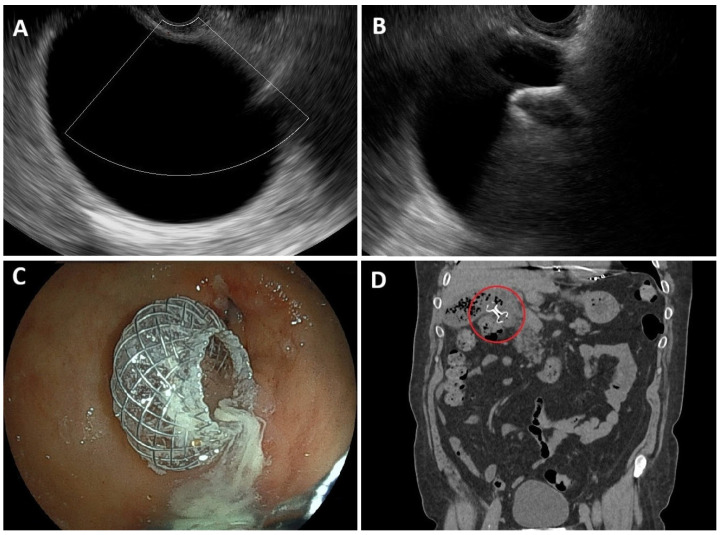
Procedural steps of EUS-guided gallbladder drainage: (A): EUS view showing a hydropic gallbladder. (B): Deployment of a lumen-apposing metal stent (LAMS) into the gallbladder. (C): Endoscopic view demonstrating the transduodenal LAMS in position. (D): Abdominal CT scan of a transduodenal LAMS in place (red circle) for gallbladder drainage.
